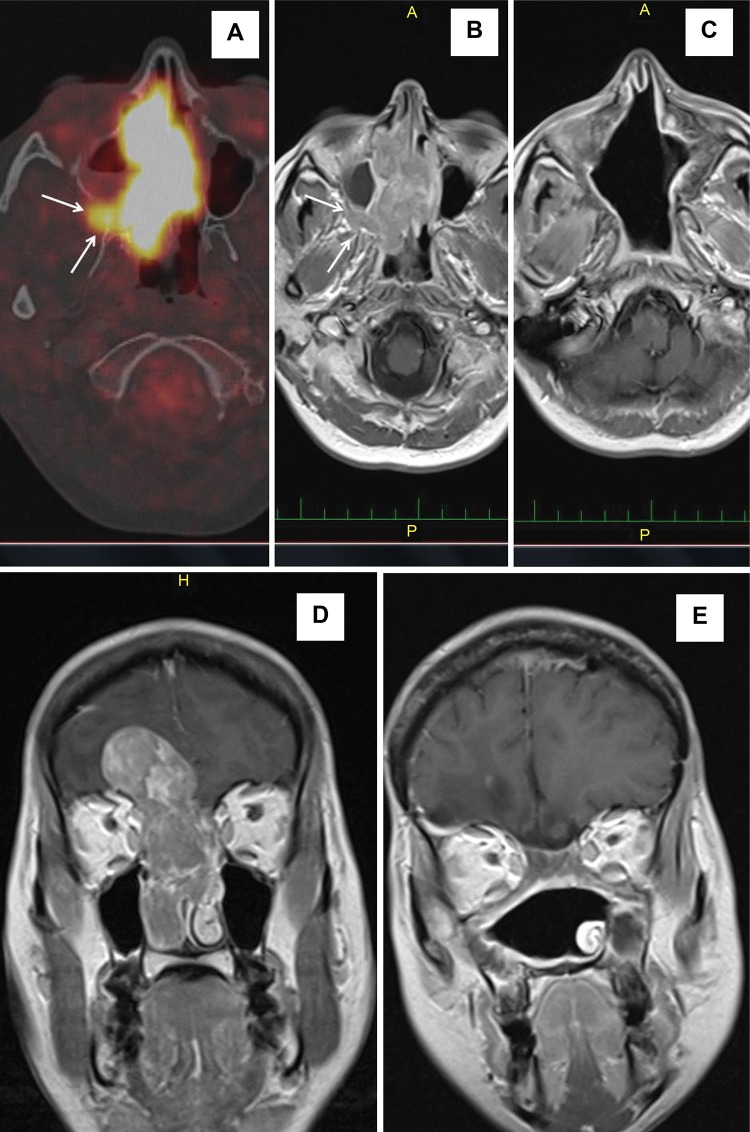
Sinonasal undifferentiated carcinoma T4b, case P13. Axial PET/CT (A) and axial (B) and coronal (D) MRI T1-weighted with gadolinium contrast images showing a mass in the bilateral nasal cavities, bilateral ethmoid sinuses, right maxillary and sphenoid sinuses, and right pterygopalatine fossa *(arrows)* with an intracranial portion with brain infiltration. Axial (C) and coronal (E) MRI T1-weighted scans with gadolinium contrast demonstrating no evidence of disease two years after surgery and postoperative chemoradiotherapy.
